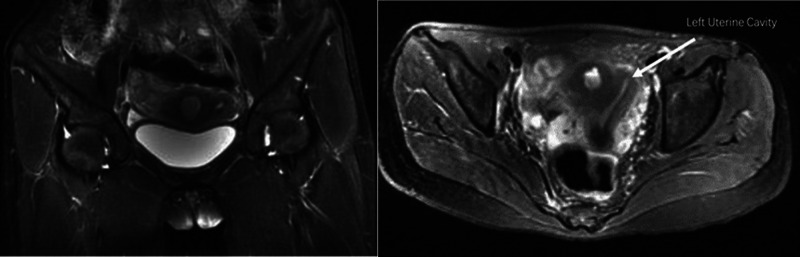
Preoperative pelvic magnetic resonance images.
